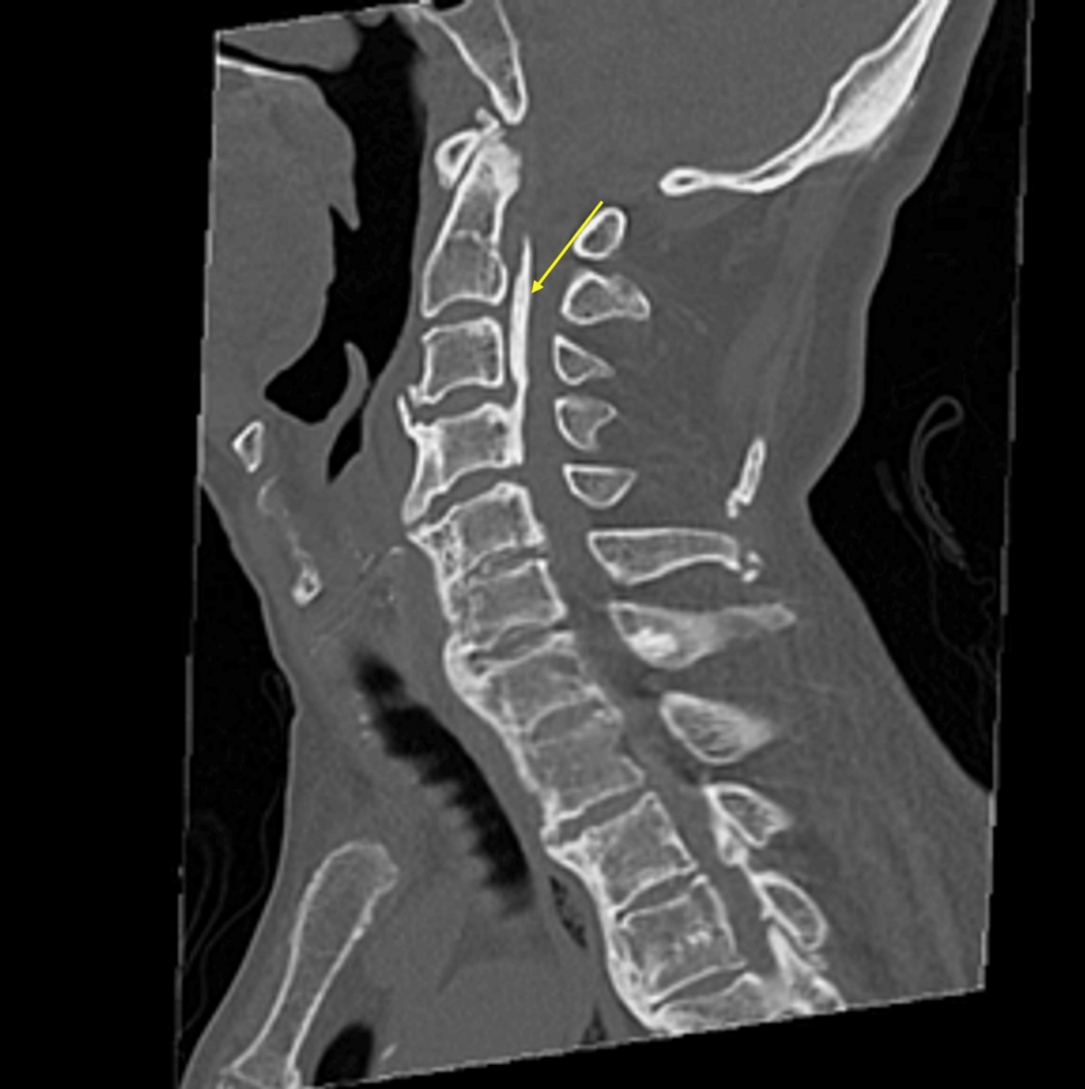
Cervical CT findings. Cervical CT shows calcification of the posterior longitudinal ligament (arrow). CT, computed tomography.
